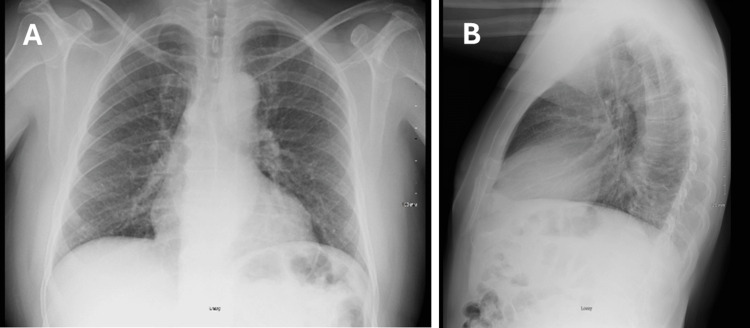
Chest X-ray demonstrating nonspecific interstitial infiltrates
